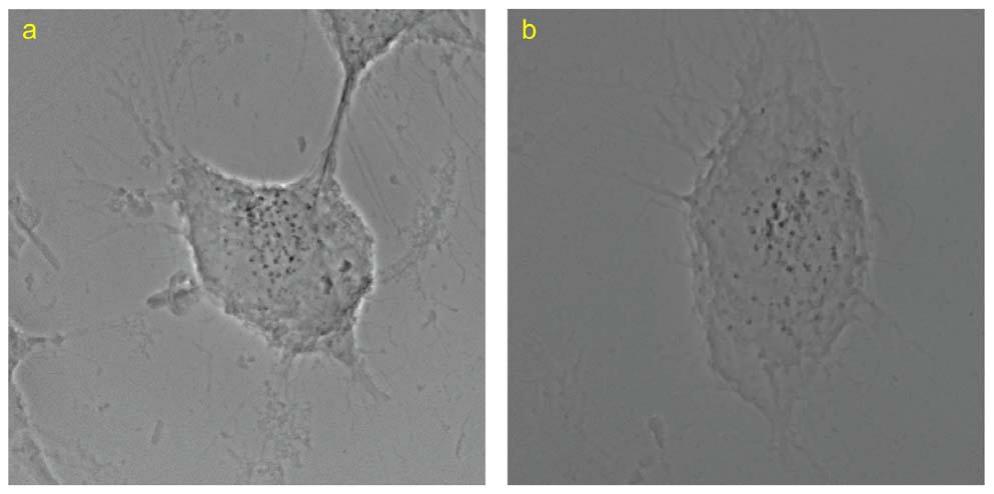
X-ray projection images of HeLa cells. 140 synchrotron X-ray projection images were acquired for HeLa1 and HeLa2. The size of each image is 1024×1024 pixels, and the rotation angle between two projection images is
